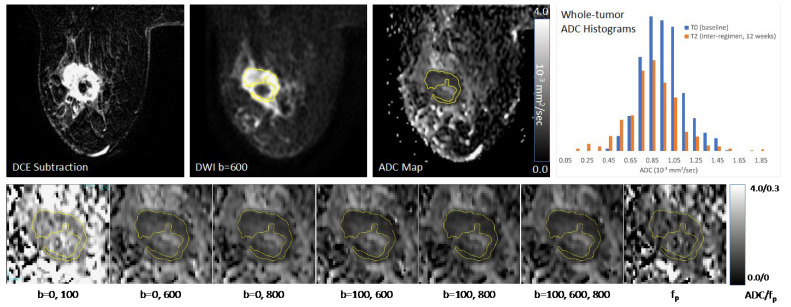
Sample images and diffusion maps from one non-pCR patient at baseline. The top row shows matched slices from the dynamic contrast-enhanced (DCE) early subtraction image, the diffusion-weighted image (DWI), and the quantitative apparent diffusion coefficient (ADC) map. Histograms are shown for the whole-tumor ADC values for this pre-treatment (T0) scan and the patient’s mid-treatment timepoint (T2) MRI. The subject showed a steady decrease in tumor volume both on DCE and DWI, but no recovery towards normal tissue ADC values was observed for the mean ADC. The bottom row shows the tumor region ADC maps for all *b*-value combinations (units 10^−3^ mm^2^/s) plus the *f_p_* map.
